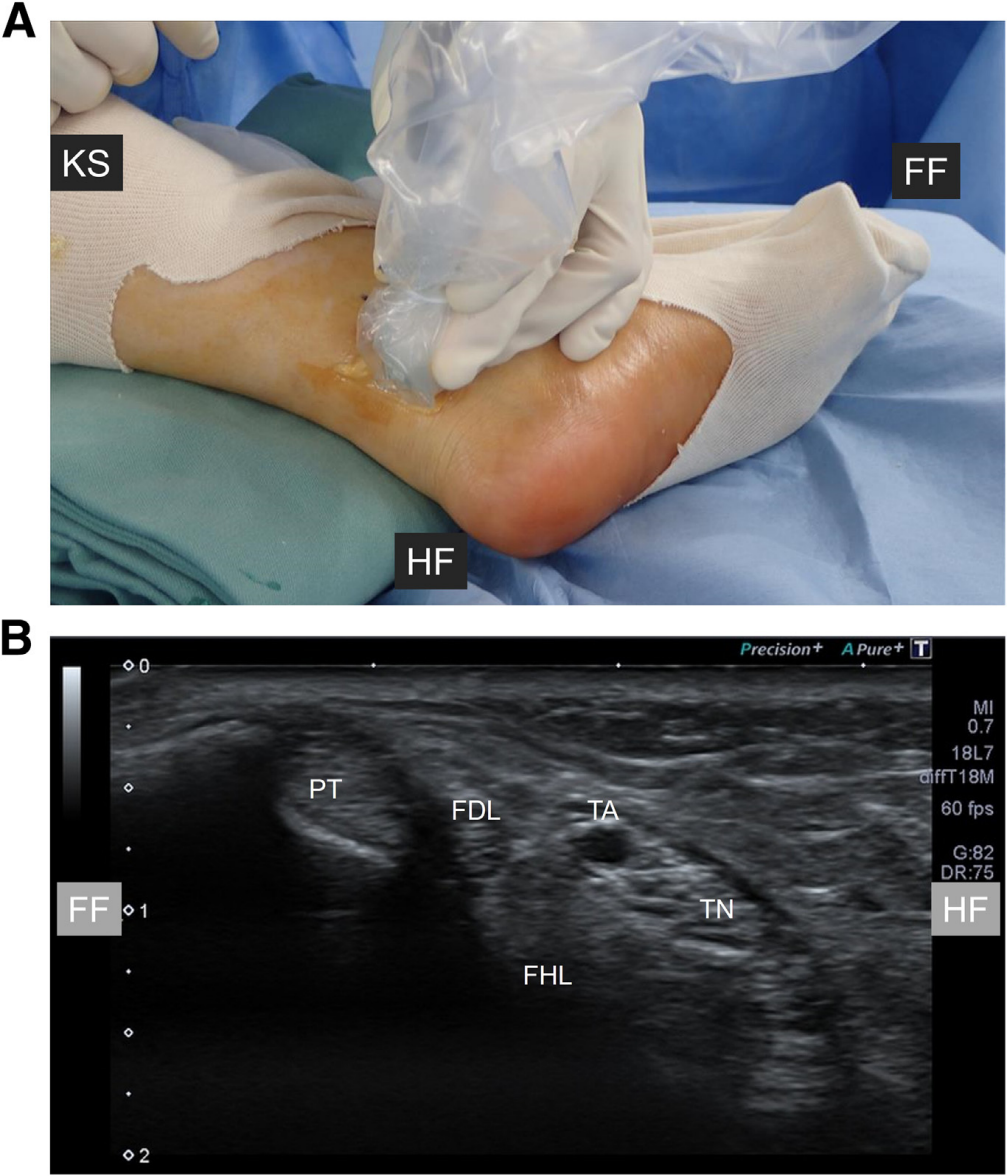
Preoperative setup and ultrasonographic anatomy of medial ankle. (A) The patient is placed in the supine position with the operative leg in the figure-of-4 position. A high-frequency linear ultrasound probe is placed posterior to the medial malleolus of the left ankle. (B) Short-axis ultrasonographic image identifying key anatomic structures. (FDL, flexor digitorum longus tendon; FF, forefoot side; FHL, flexor hallucis longus tendon; HF, hindfoot side; KS, knee side; PT, posterior tibial tendon; TA, tibial artery; TN, tibial nerve.)
